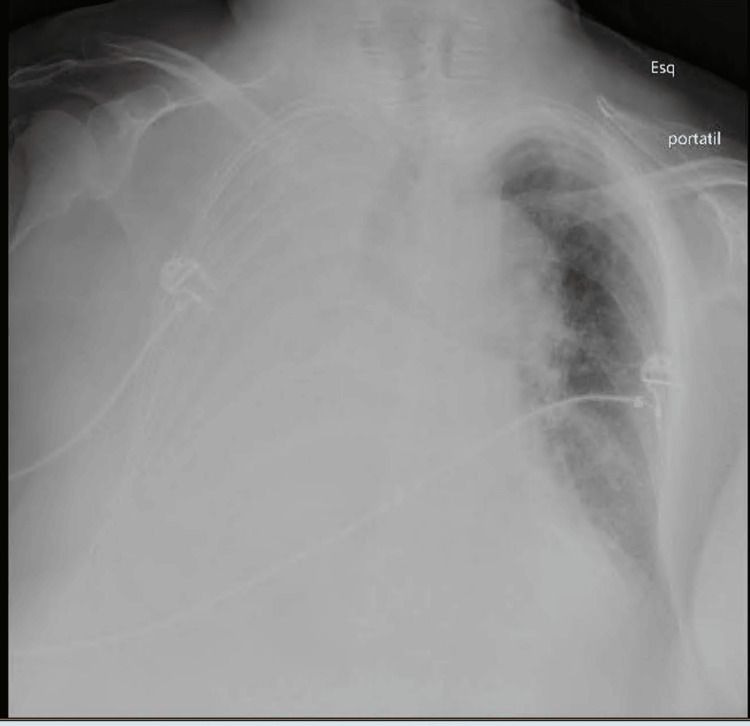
Anteroposterior chest X-ray showing extensive right pleural effusion.
